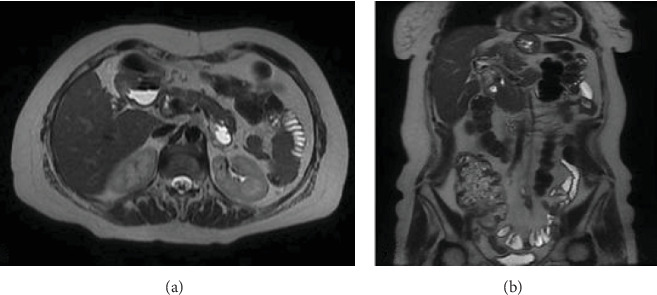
(A) T2 sequence magnetic resonance image in axial projection shows the right liver without signs of recurrence of the cystic lesion, (B) T2 sequence magnetic resonance image in coronal projection.
